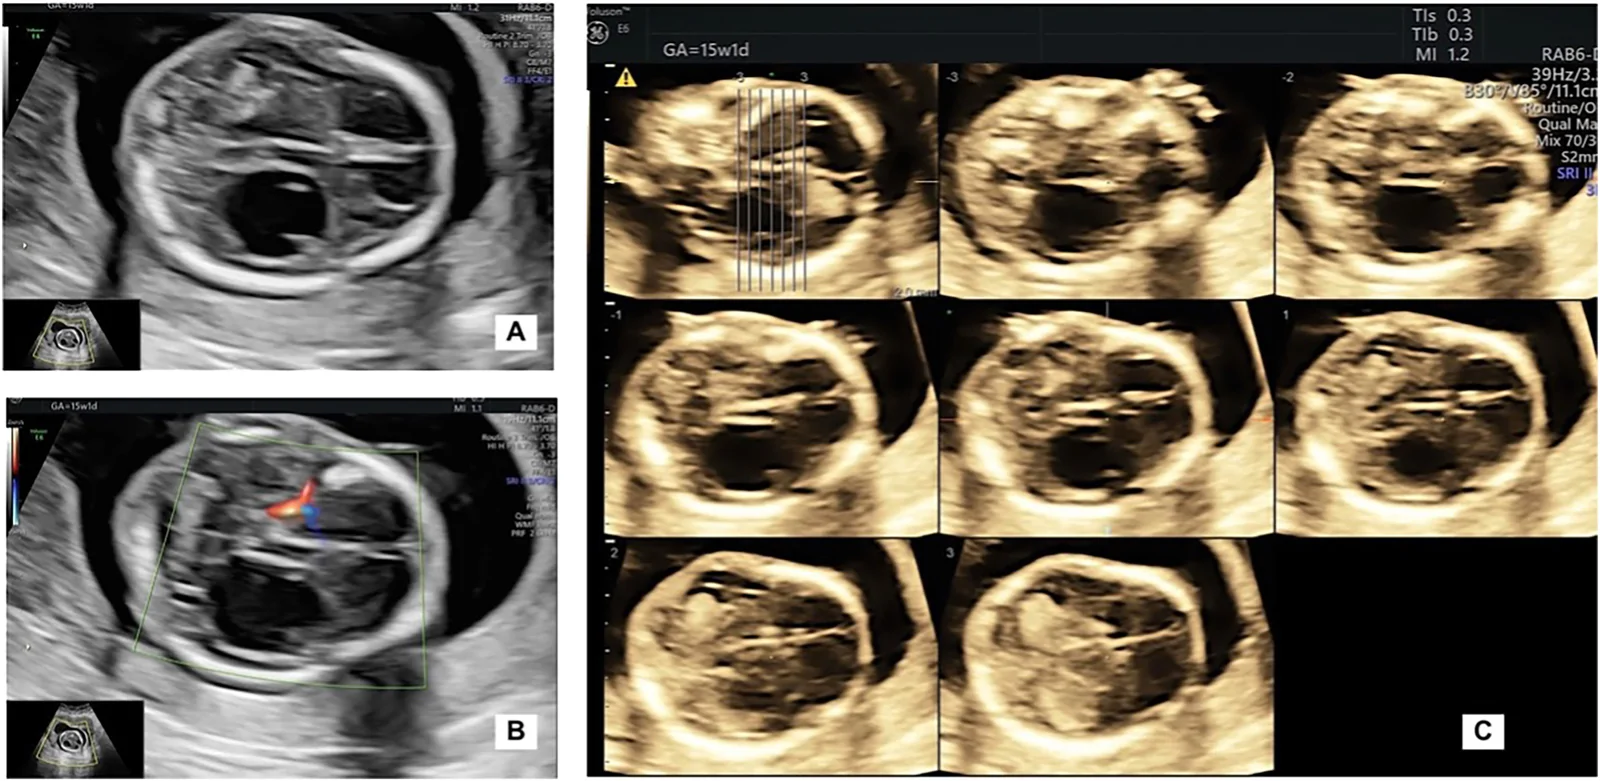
Fetal neurosonography findings at 15 weeks of gestation. (A) Unilocular, anechoic cystic structure in the axial plane, close to the midline in the left hemisphere, unrelated to the lateral ventricles. (B) Demonstration of the avascular character of the same lesion using color Doppler. (C) Appearance of the unilocular structure and intracranial location of the cystic lesion in multiple sections.
